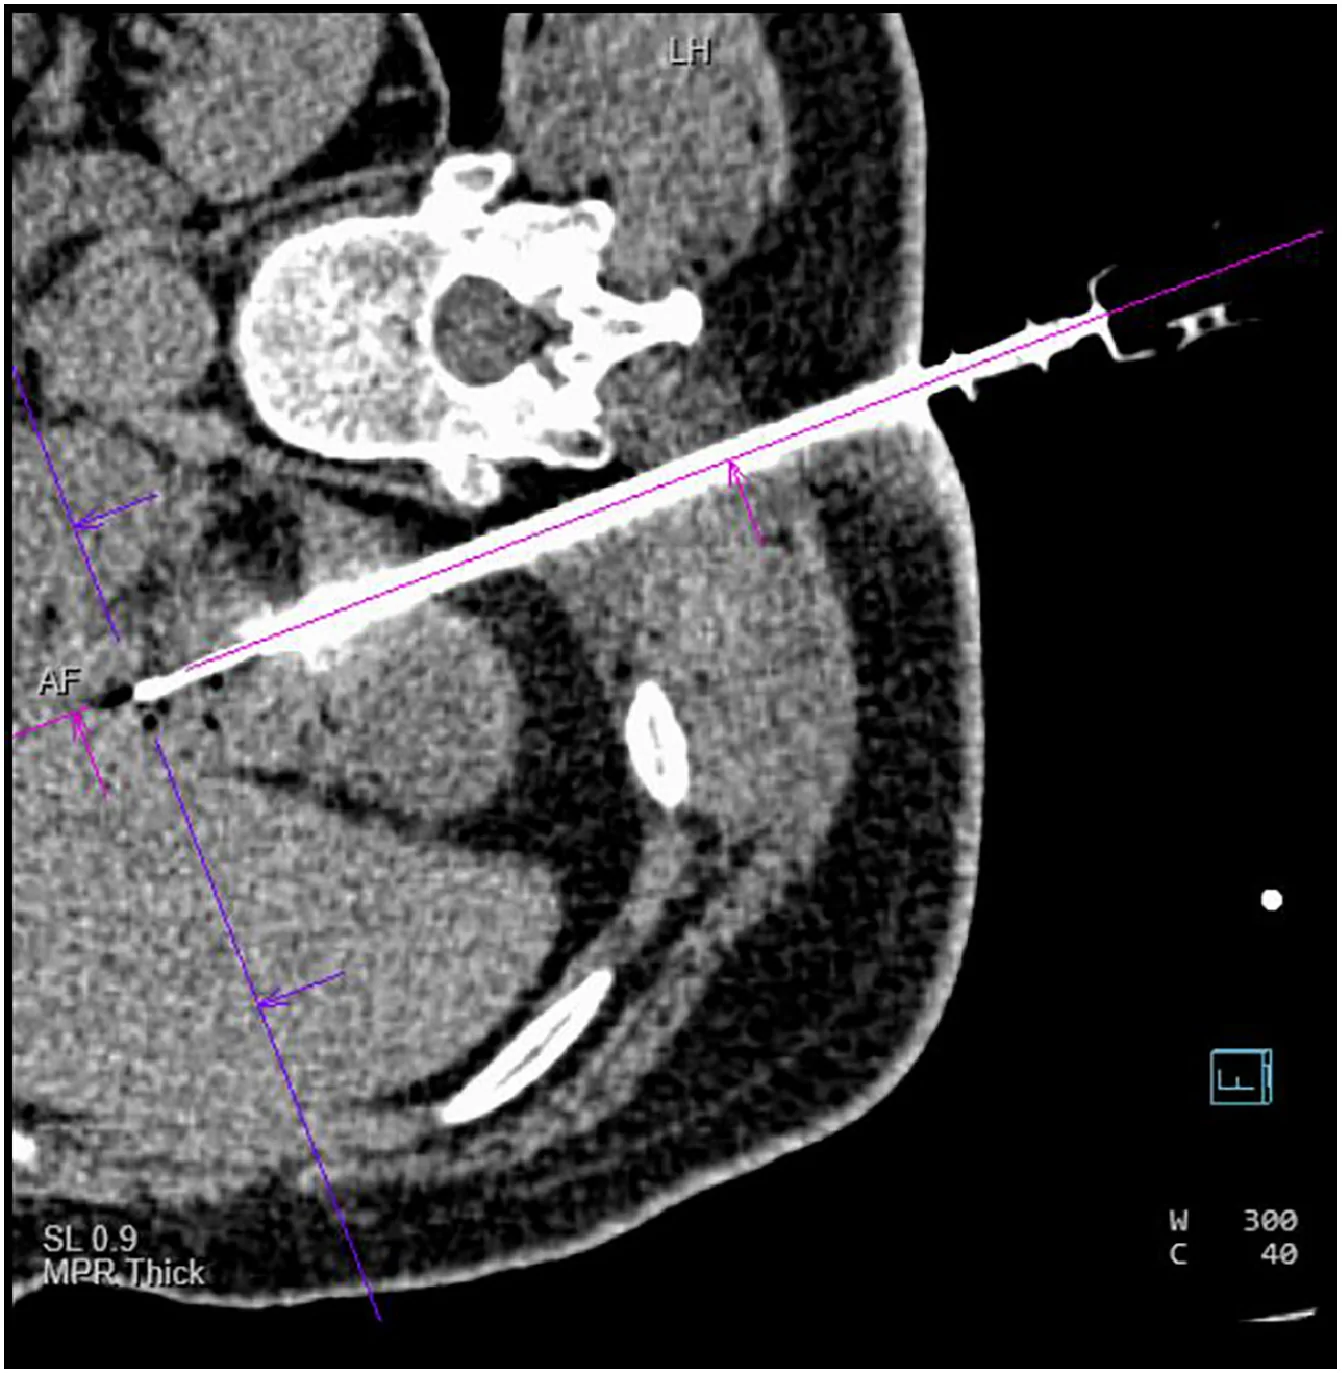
CT-guided biopsy of the right perirenal mass. Intraprocedural CT demonstrates the biopsy needle positioned along a percutaneous paravertebral approach for sampling of the lesion.
